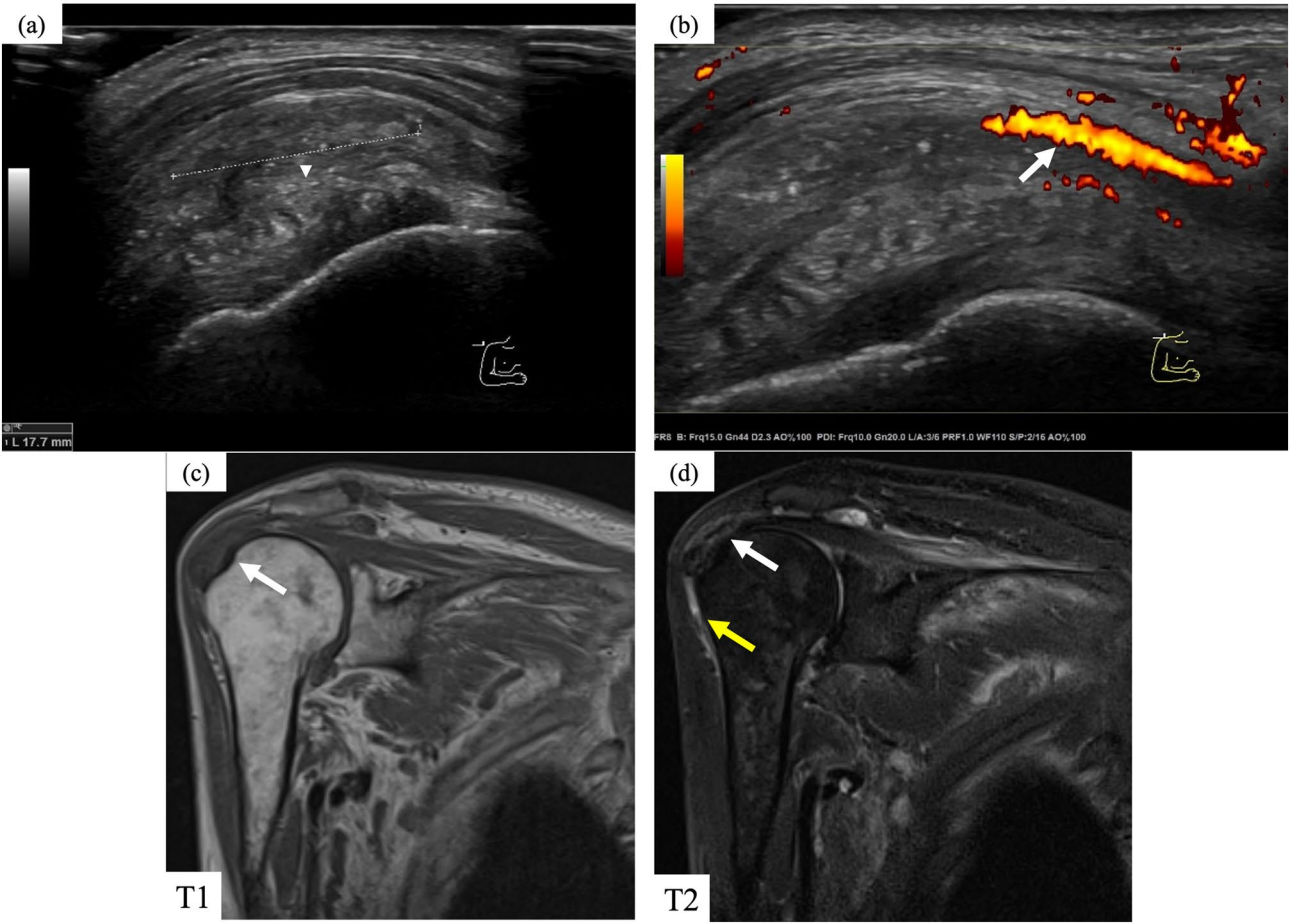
Imaging findings. a Ultrasonography of the shoulder joint showed a uniform mosaic echogenic area (white arrowhead) in the subdeltoid smooth muscle. b Doppler ultrasonography revealed increased blood flow (white arrow) around the mosaic echo area. c MRI showed an area of low intensity (white arrow) on T1-weighted images. d MRI showed low to normal intensity (white arrow) on T2-weighted images. In addition, there was an inflammatory image with high intensity (yellow arrow) on T2-weighted images. *MRI* magnetic resonance imaging
